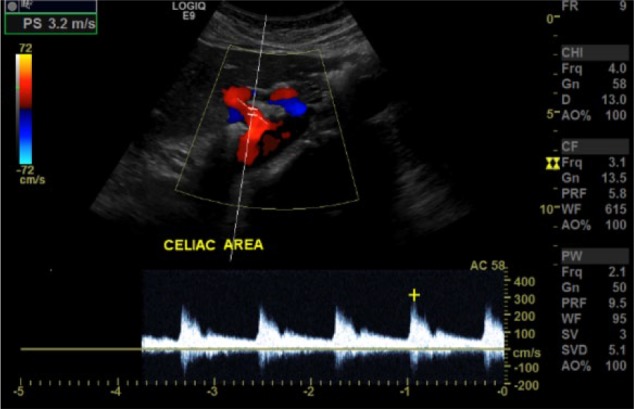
Mesenteric ultrasound of celiac artery with velocity of 320 cm/s.
